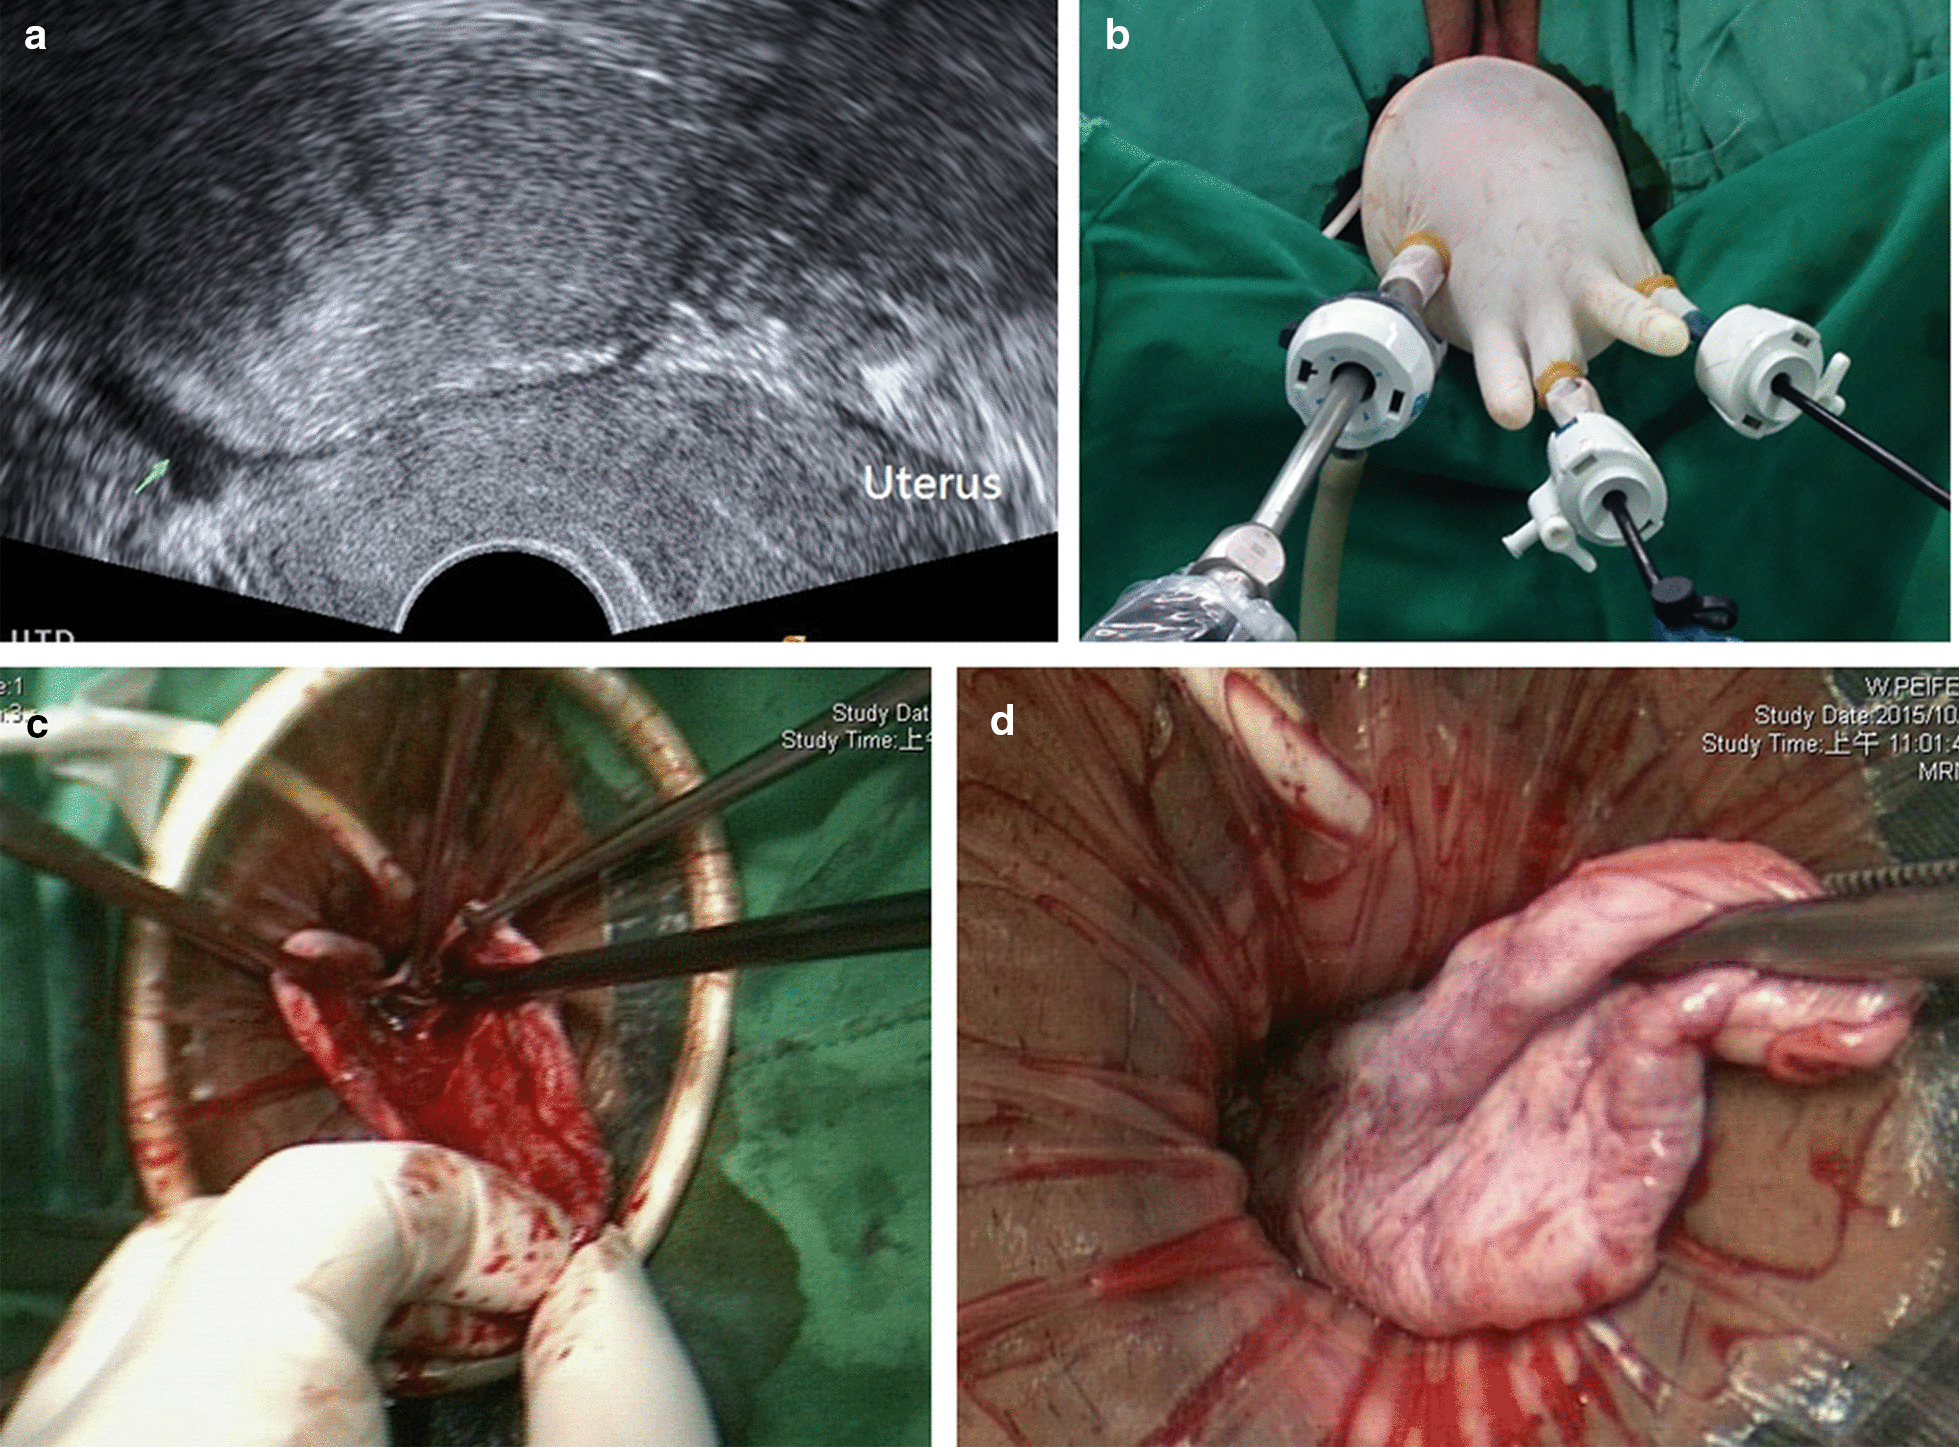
a Transvaginal ultrasonography showing a well-defined solid tumor (arrow) measuring 9.49 × 5.39 cm in the right adnexal area. b Intraoperative images showing the transvaginal natural orifice transluminal endoscopic portal. c Tisseel is applied on the inner ovarian surface defects with a dual lumen applicator. d The ovarian defect is approximated with an atraumatic forceps for 3 min
